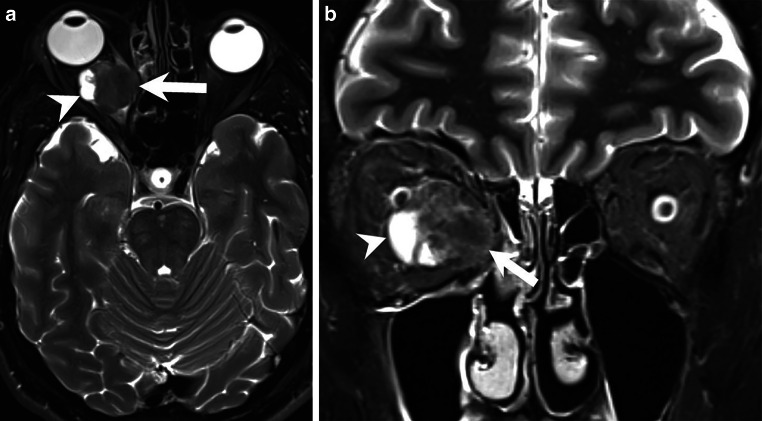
Axial (a) and coronal (b) T2-weighted images showed an intraorbital, intraconal mass (*arrows*) located medially of the optic nerve. Cystic components were presented on the lateral tumor margin (*arrowheads*). Note the right-sided exophthalmos on the axial view (a)
